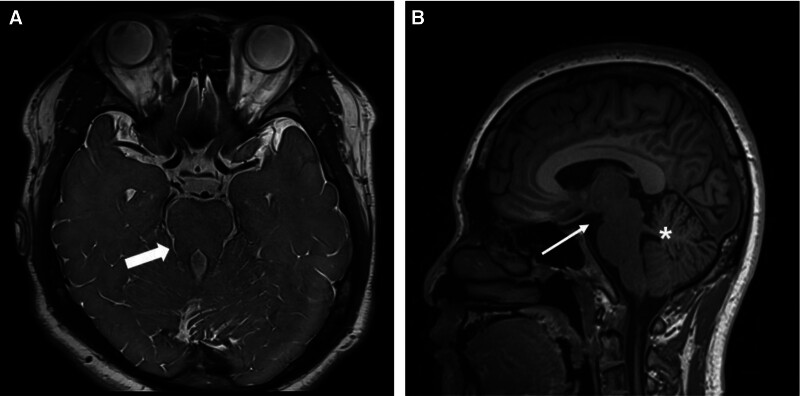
Brain magnetic resonance images. (A) An axial T2 image indicating mildly elongated superior cerebellar peduncles (thick arrow) and (B) a sagittal T2 image visualizing normal interpeduncular fossa depth (thin arrow) with no prominent vermian hypoplasia (asterisk).
